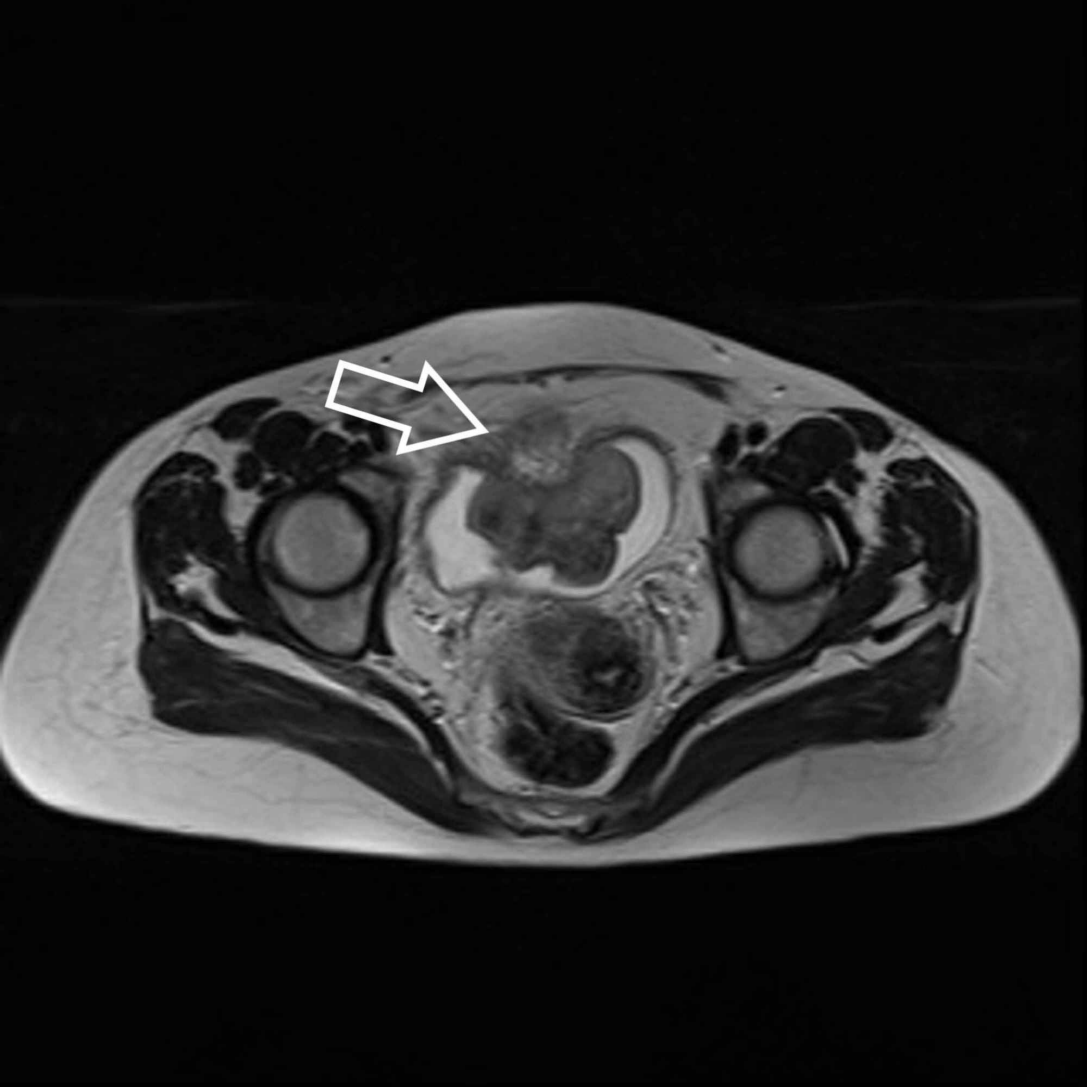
T2-weighted MRI shows protruding mass with extravesical extension (arrow) at the anterior bladder wall. MRI, magnetic resonance imaging
